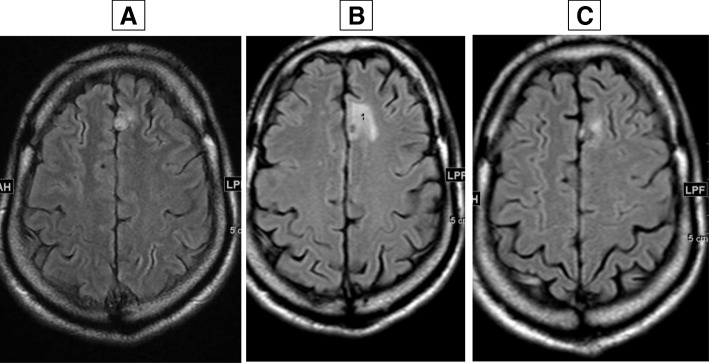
Brain magnetic resonance imaging of Case 1. a July 2016, b December 2016, c May 2017 4 months under cabozantinib
